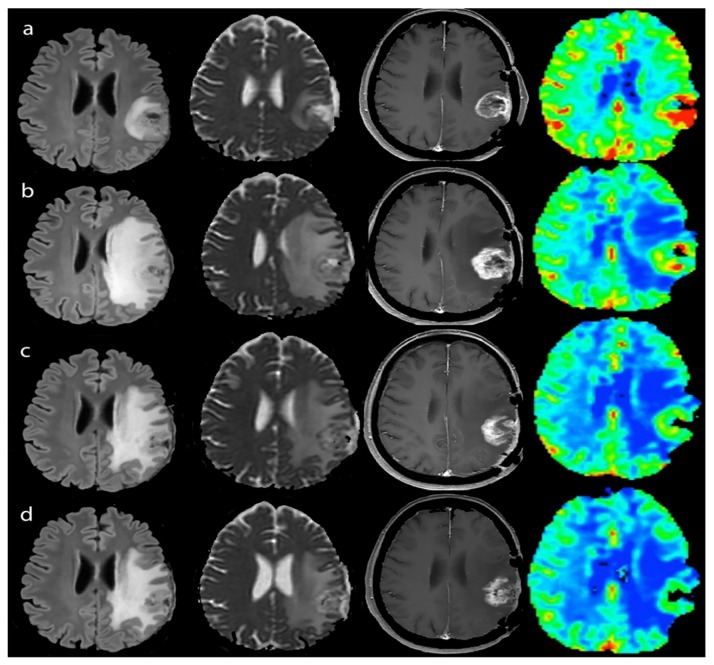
Pseudoprogression during immunotherapy of a LowNK and hypermethylated case - Left to right: FLAIR, ADC map, T1-enhanced and CBV map. (a) Jan 2015, MRI-pre, after surgery and radio-chemotherapy (steroid dose 2 mg Dexamethazone, clinical condition stable): GBM showing contrast enhancement (T1) and hyper-perfusion with high CBV (red-colored), slight edema (FLAIR) and non-homogeneous ADC restriction as in hypercellularity; (b) Mar 2015, MRI-2 mo during immunotherapy (no steroid therapy, clinical condition stable): enlargement of enhancing volume and of edema with mass effect and reduction of hyper-perfusional intensity (yellow to green CBV with small red spots) and persistent ADC un-homogeneity; (c,d) Jun and Aug 2015, MRI-4 and -6 mo after immunotherapy (no steroid therapy clinical condition stable): reduction of enhancing volume, edema and CBV intensity (green) and volume of hyper-perfusion with less restricted ADC.
